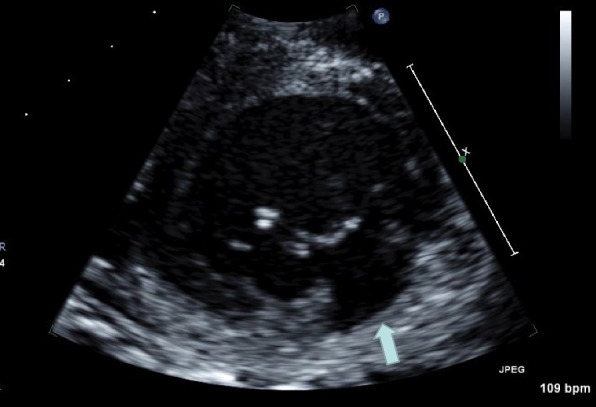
Transthoracic echocardiography before the second surgery. The shorting of the posterior leaflet is observed especially on the median side (arrow)
